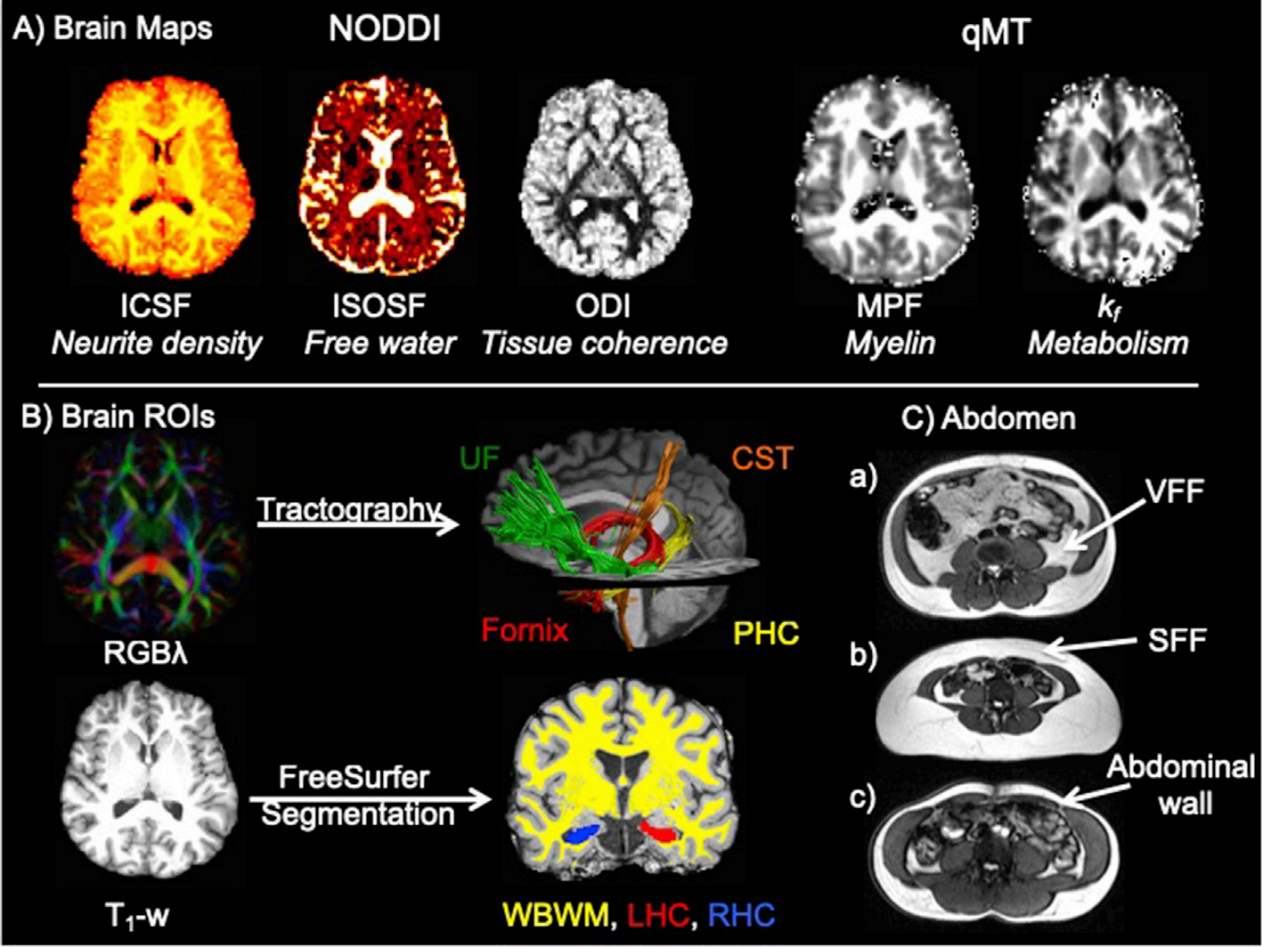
A) displays the MRI modalities and maps from dual-shell high angular resolution imaging (HARDI) and quantitative magnetization transfer (qMT) imaging. HARDI data were modelled with neurite orientation dispersion and density (NODDI) yielding maps of intracellular signal fraction (ICSF), isotropic signal fraction (ISOSF) and orientation density index (ODI). qMT based maps were the macromolecular proton fraction (MPF) and the forward exchange rate *k*_f_. B) Mean indices of the metrics were extracted from the left hippocampus (LHC) (red), right hippocampus (RHC) (blue), whole brain white matter (WBWM) mask (yellow), fornix (red), parahippocampal cinguli (PHC) (yellow), uncinate fasciculi (UF) (green) and corticospinal tract (CST) (orange). Hippocampi and WBWM were segmented from T_1_-weighted images with FreeSurfer version 5.3 and fornix, PHC, UF and CST were reconstructed with damped-Richardson Lucy spherical deconvolution (dRL) based deterministic tractography on colour coded principal direction maps (RGBλ). C) Examples of abdominal images from a) a 51 years old male with larger visceral (VFF) than subcutaneous fat area fraction (SFF), b) a 52 years old female with larger SFF than VFF and c) a 60 years old female with low SFF and VFF. All abdominal images were acquired with the same field of view of 480 × 390 mm.
